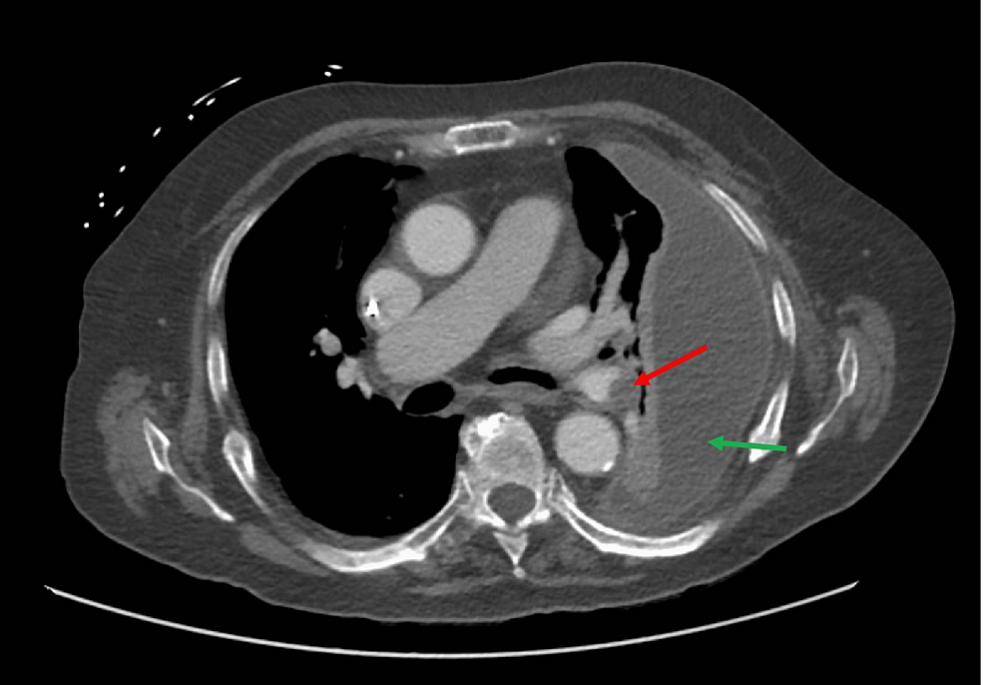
Computed tomography of the chest demonstrating pulmonary thromboembolism in the left main pulmonary artery (red arrow) and large loculated left-sided pleural effusion (green arrow)
